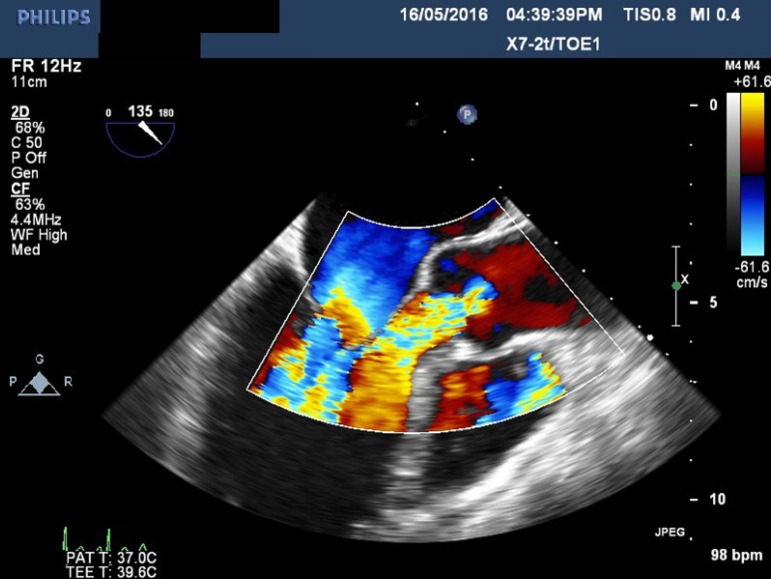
Transesophageal echocardiogram showing massive aortic regurgitation.
